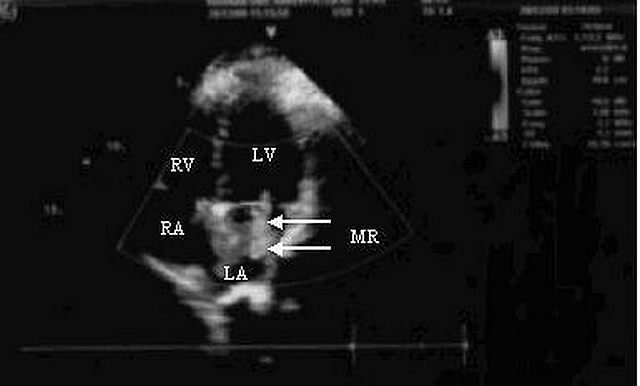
Transthoracic echocardiogram (TTE) showing moderate to severe mitral regurgitation (MR) with an estimated regrgitant fraction of 45% (white arrows).
